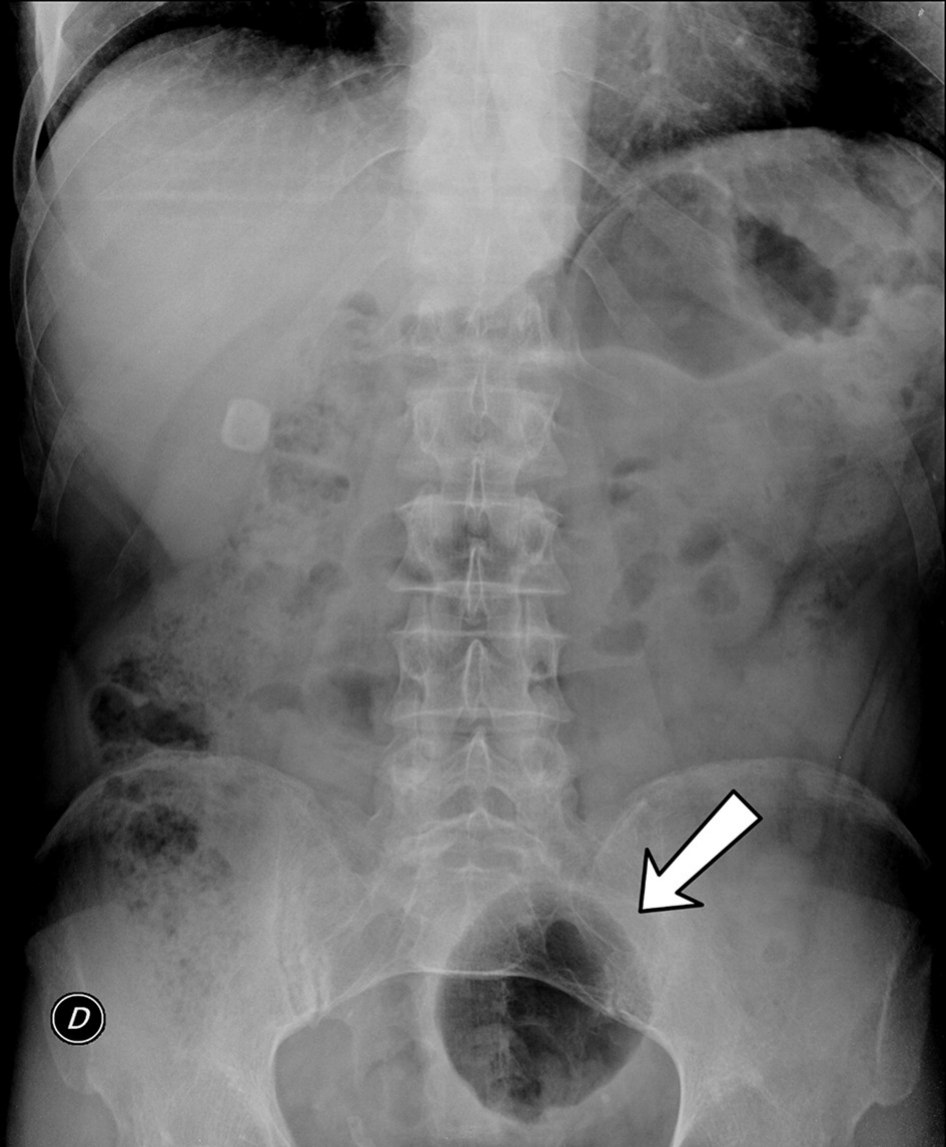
Abdominal X-rays showing a large gas-filled cavity in the lower abdomen (arrow).
